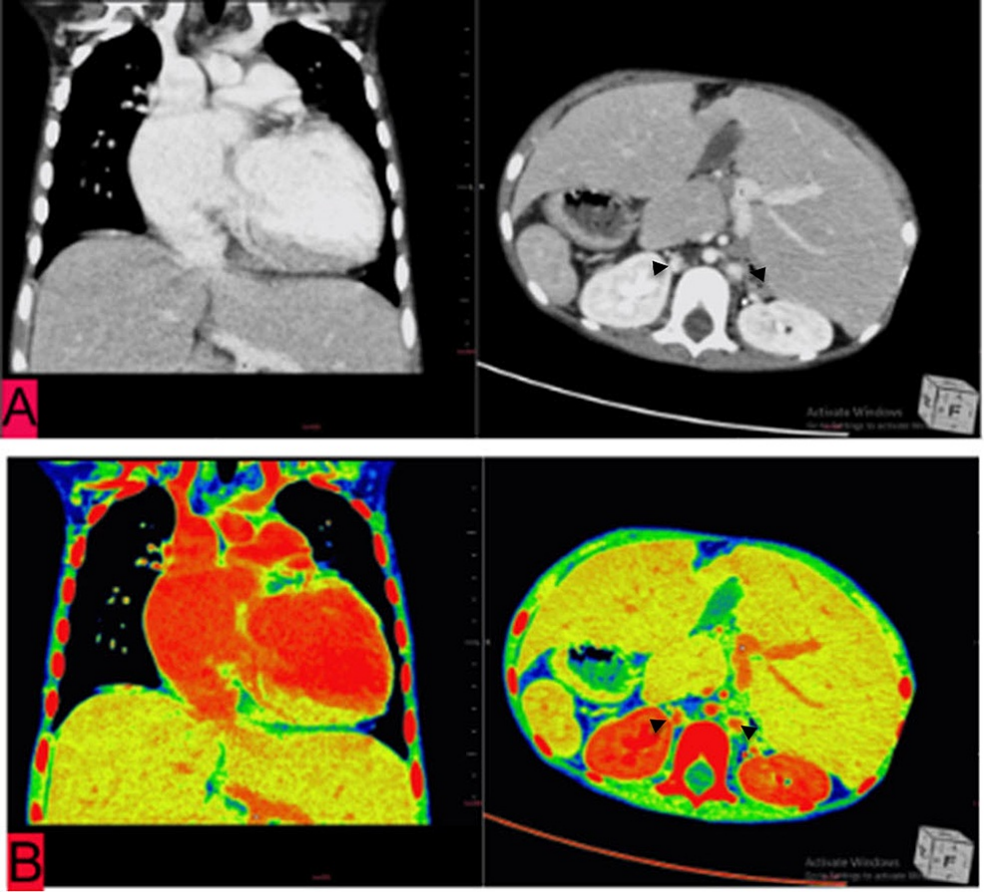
Axial and coronal sections of chest CT of a five-year-old child suffering from heterotaxy syndrome reconstructed on abdominal window (HU) (A) and then on PET-CT window (B) for better visualization revealing a central aorta flanked by a hemiazygos vein on either side (black arrowheads), which also reveals the left branch of portal vein PET-CT, Positron emission tomography–computed tomography.
